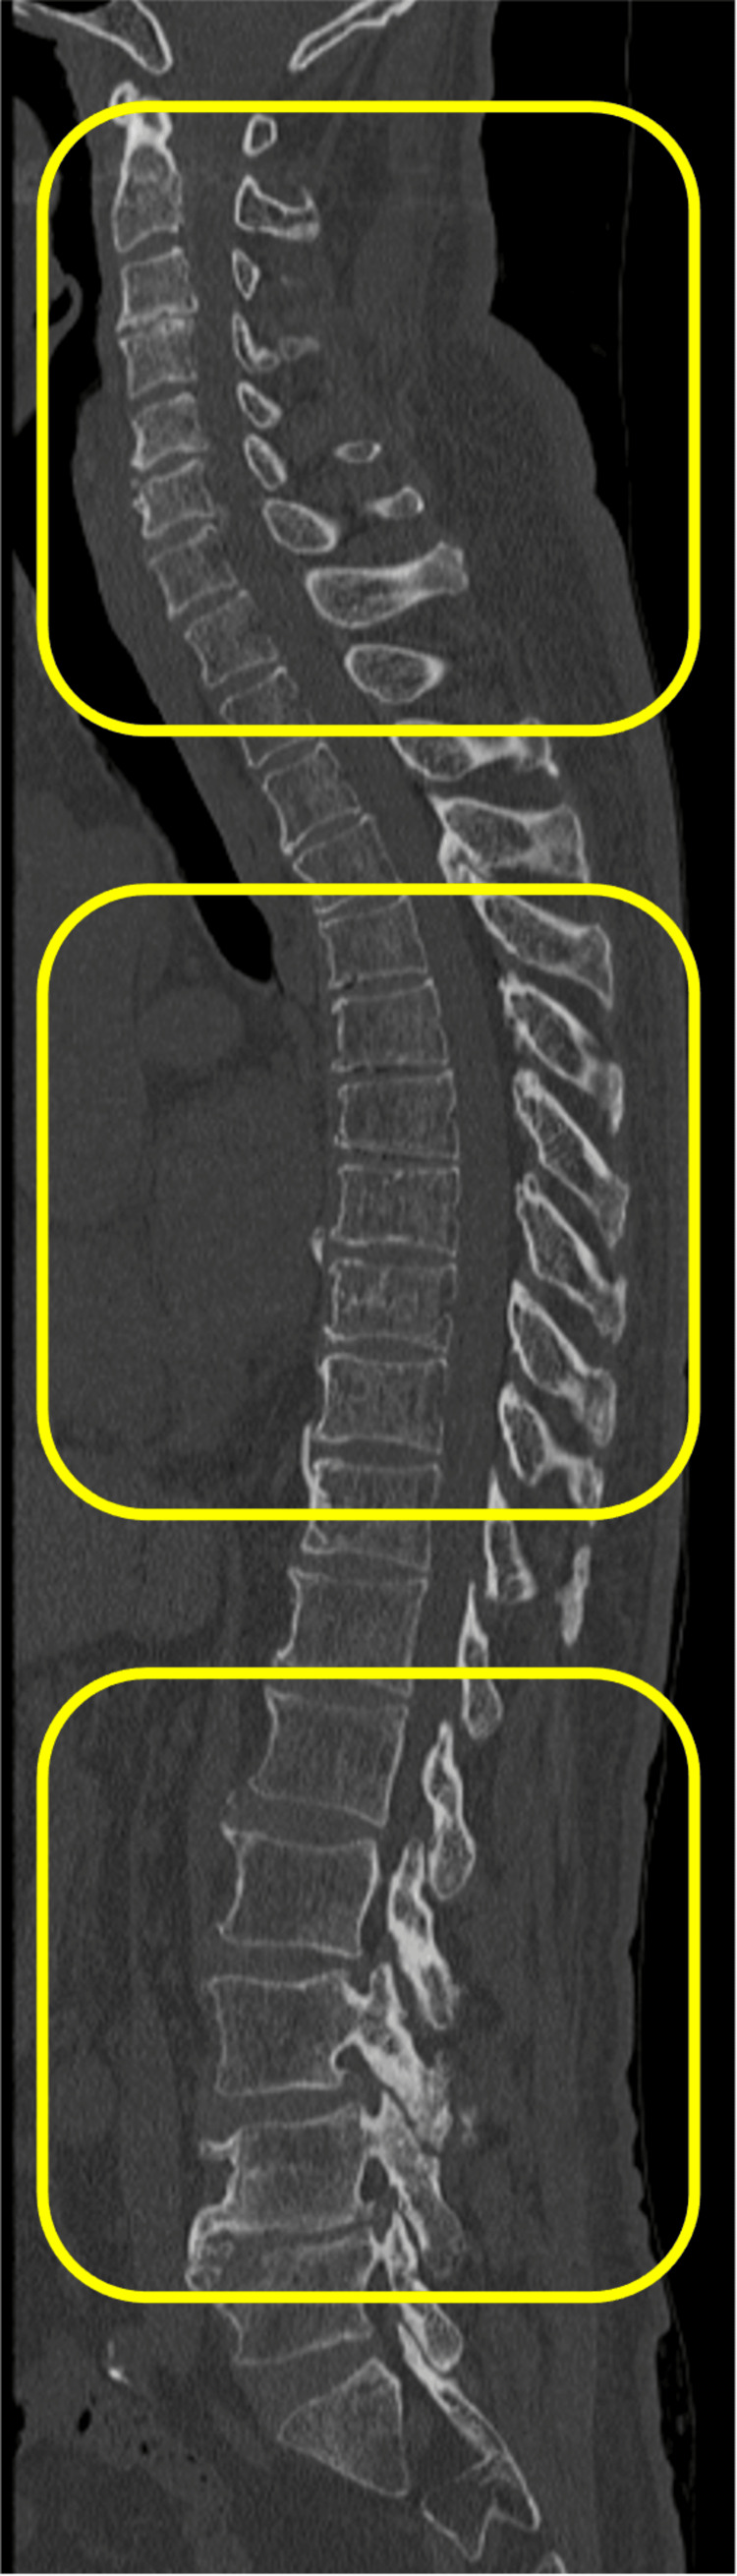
Measurement of cervical, thoracic, and lumbar spine skin thickness using sagittal whole-spine CT. The average skin thickness at the cervical (4-5, C5-6, C6-7 disc levels), thoracic (T7-8, T8-9, T9-10 disc levels), and lumbar (L2-3, L3-4, L4-5 disc levels) is defined as skin thickness.
